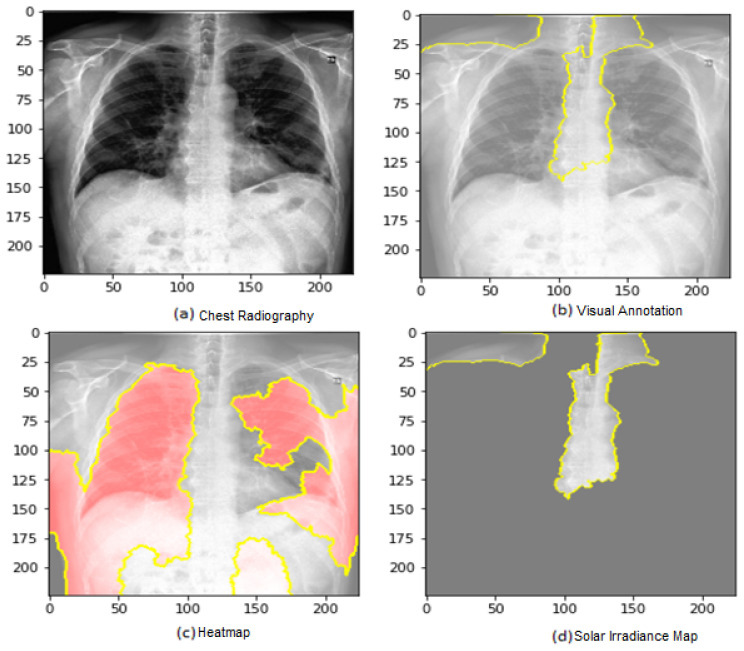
Explainability of X-ray images in the fourth dataset.
